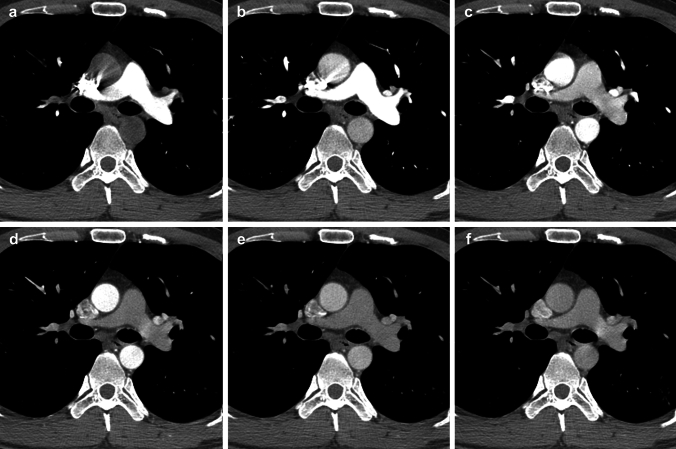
Multiphase contrast-enhanced chest CT images with six phases are shown. Each phase was obtained at a 6 s, b 11 s, c 16 s, d 21 s, e 26 s, and f 31 s after the beginning of contrast agent injection. Images a and b are included in the image set of CT angiography for the pulmonary artery (CTA-P), and images c–e in CT angiography for the systemic artery (CTA-S). The serial assessment of CTA-P and CTA-S (CTA-PS) includes images a–e. Image f the phase without difference between pulmonary arteries and thoracic aorta regarding CT values, is excluded from the three image sets. CT, computed tomography
